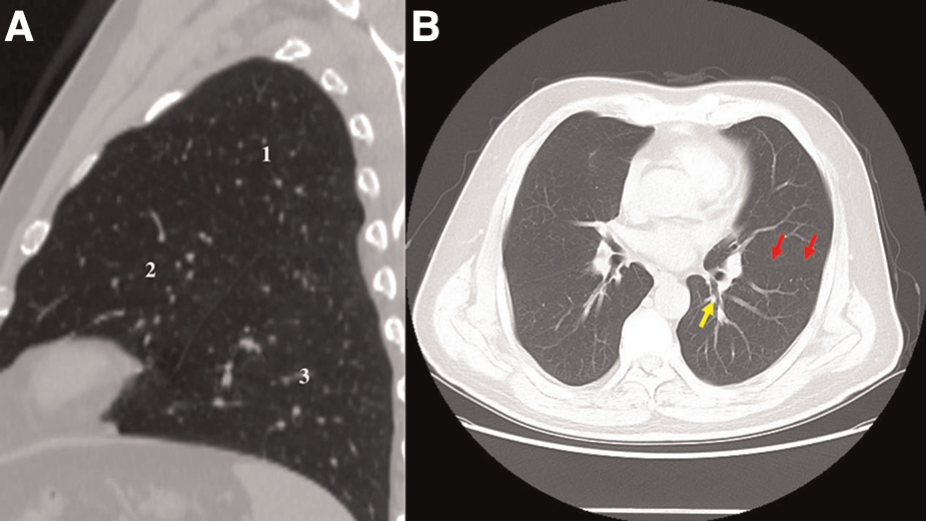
(A) CT image before the surgery shows the three lobes of the left lung: 1, accessory lobe (S1 + 2); 2, the rest upper lobe; 3, lower lobe. (B) CT image before the surgery. The red arrow refers to true oblique fissure. The yellow arrow refers to the true S6 bronchus.
